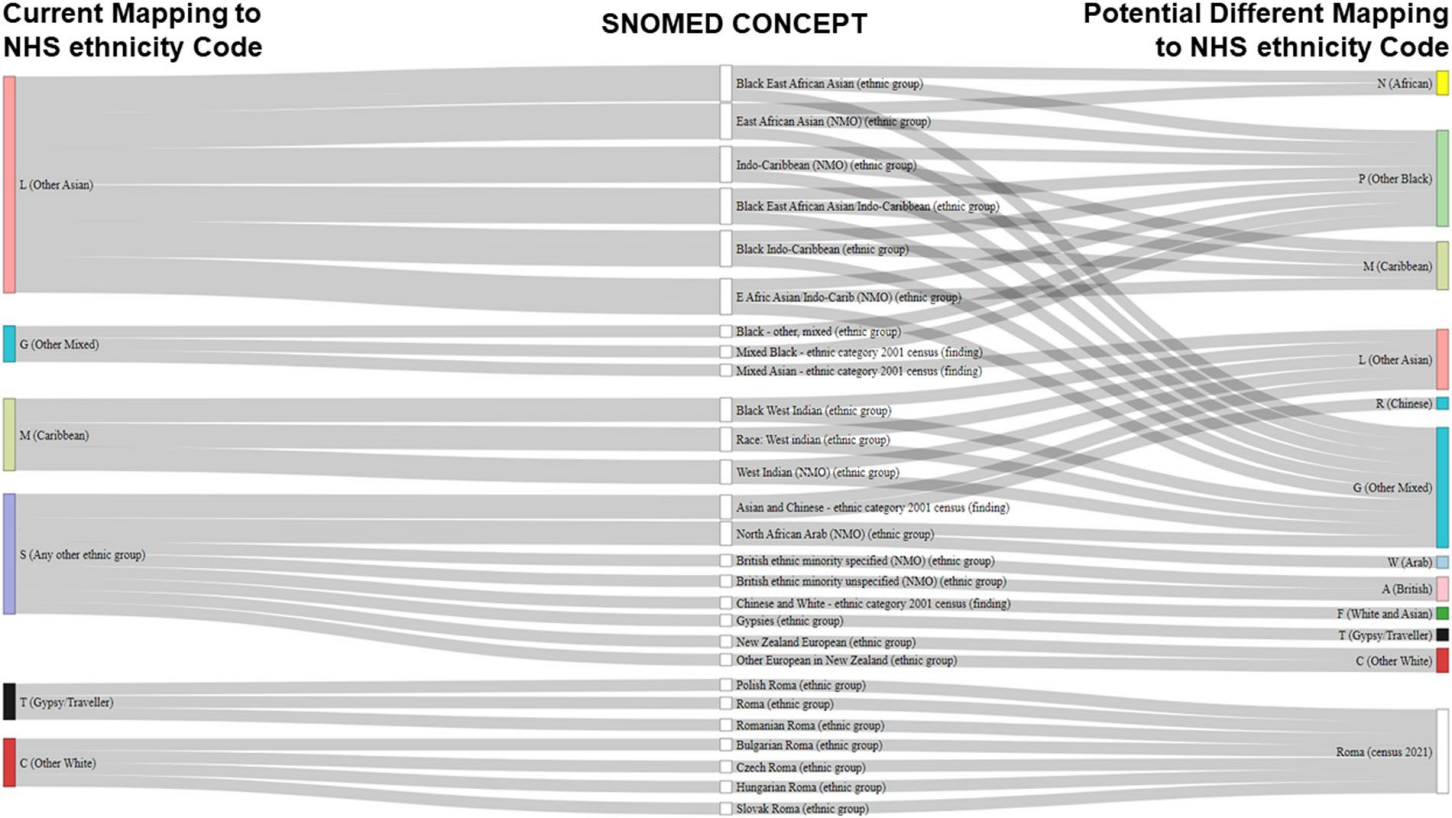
Sankey plot showing potential discrepancies between SNOMED concepts and NHS ethnicity codes mapping. Abbreviations: NHS, National Health Service in the UK; SNOMED, SNOMED-CT records containing ethnicity concepts.
